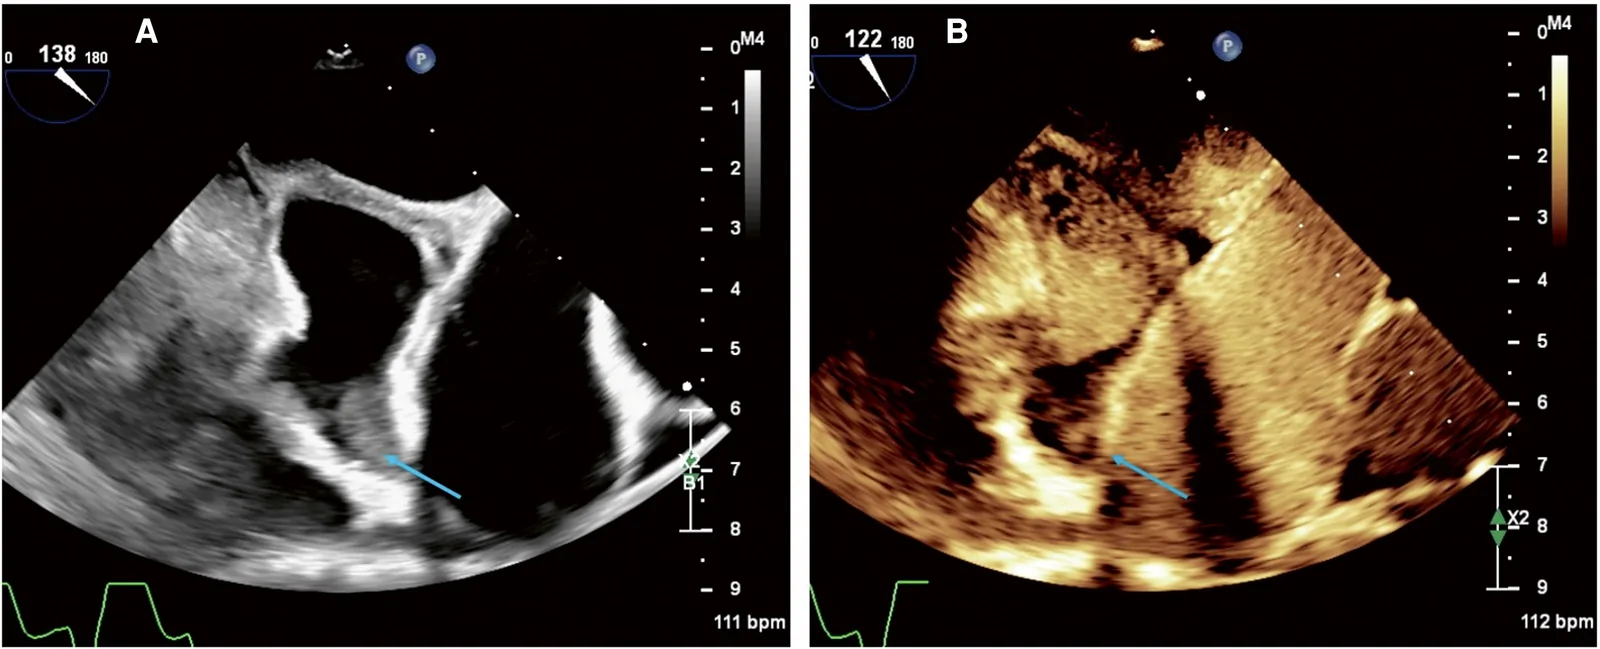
TEE images prior to DCCV. (*A*) Mid-oesophageal view demonstrating a filling defect within the LAA, suspicious for thrombus (arrow). (*B*) Contrast-enhanced TEE confirming the presence of thrombus with lack of contrast opacification in the appendage (arrow). TEE, transoesophageal echocardiogram; LAA, left atrial appendage; DCCV, direct current cardioversions.
